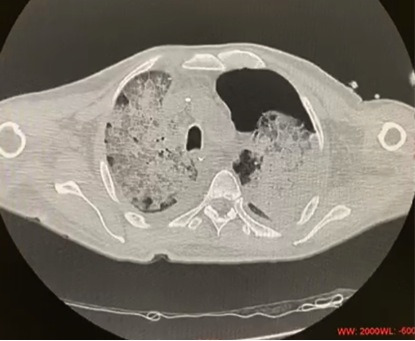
Chest CT scan showing ground glass opacities and the crazy-paving pattern in the lungs.
